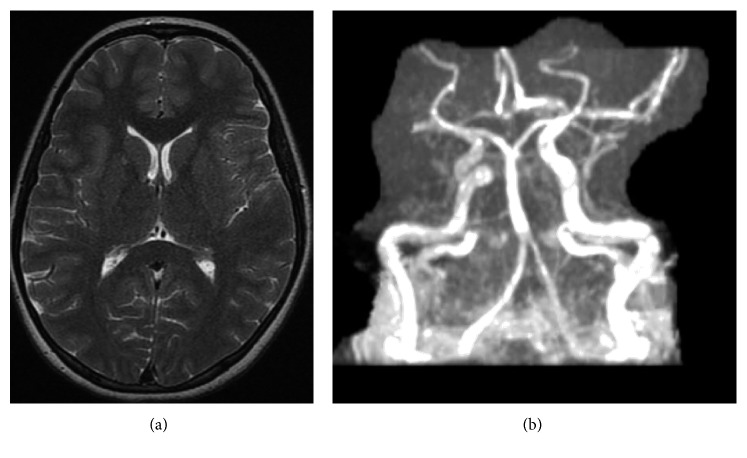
(a) MRI brain within normal limits; (b) MRA brain within normal limits.
